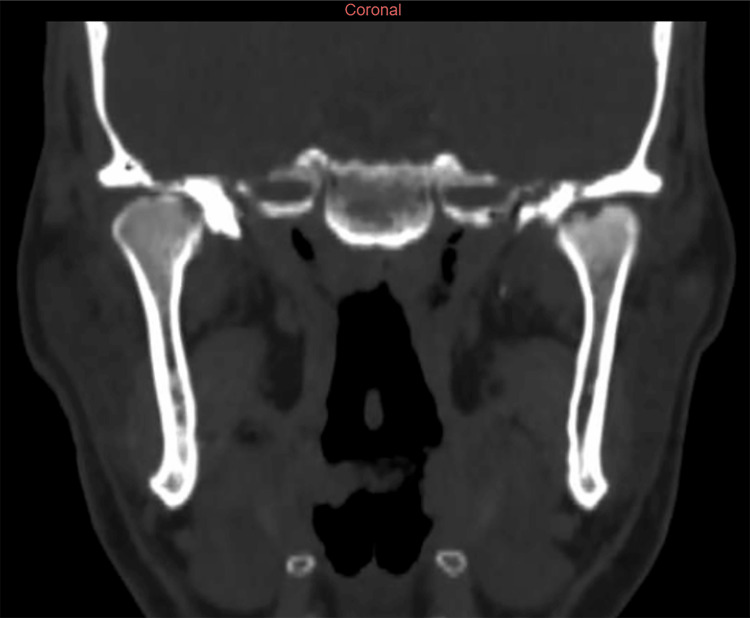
CT scan image of the patient’s TMJ showing bilateral condylar and temporal cortical erosion with joint space narrowing TMJ: Temporomandibular joint
